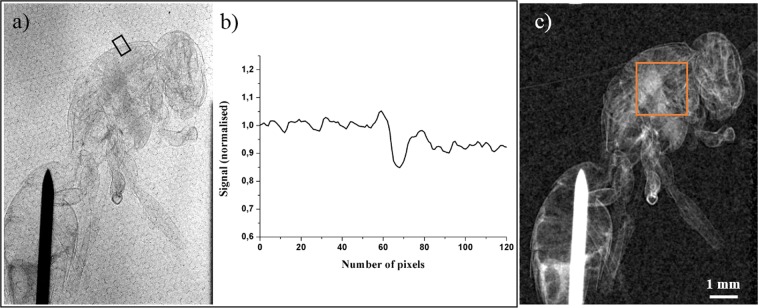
(a) Raw x-ray phase contrast image of the wasp acquired at I = 1.3 × 10^19^ W/cm^2^ and with 200 x-ray pulses. (b) Line profile of the signal perpendicular to the edge of a thorax zone. (c) Corresponding x-ray retrieved phase image.
